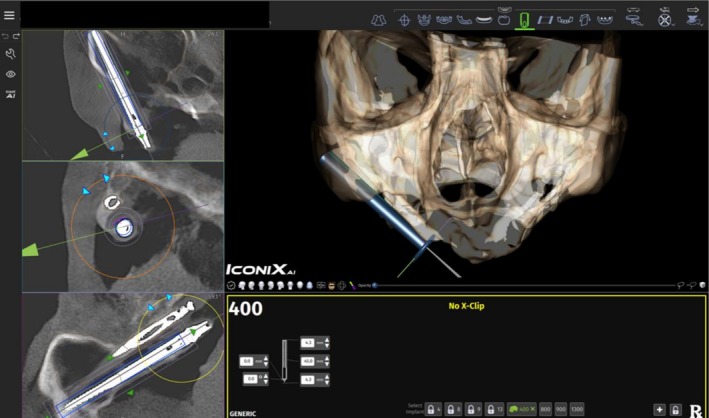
Accuracy evaluation: Implant library geometries were aligned in the postoperative cone beam computed tomography (CBCT) to the respective images of the placed implants.
